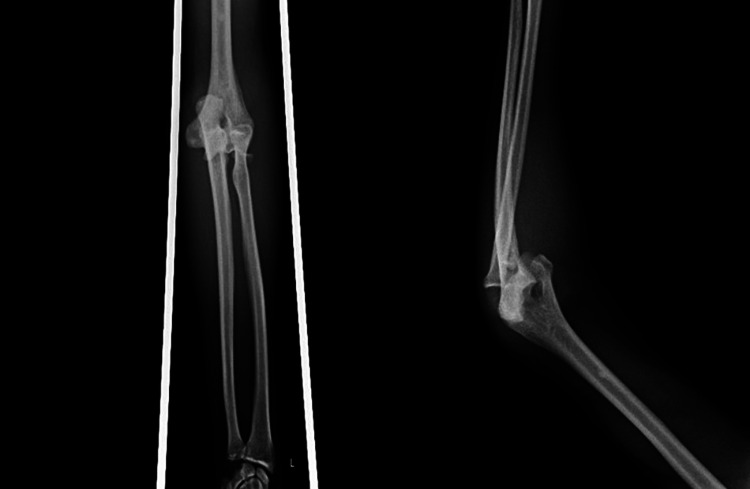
X-ray anteroposterior and a lateral view showing posterior dislocation of the elbow
